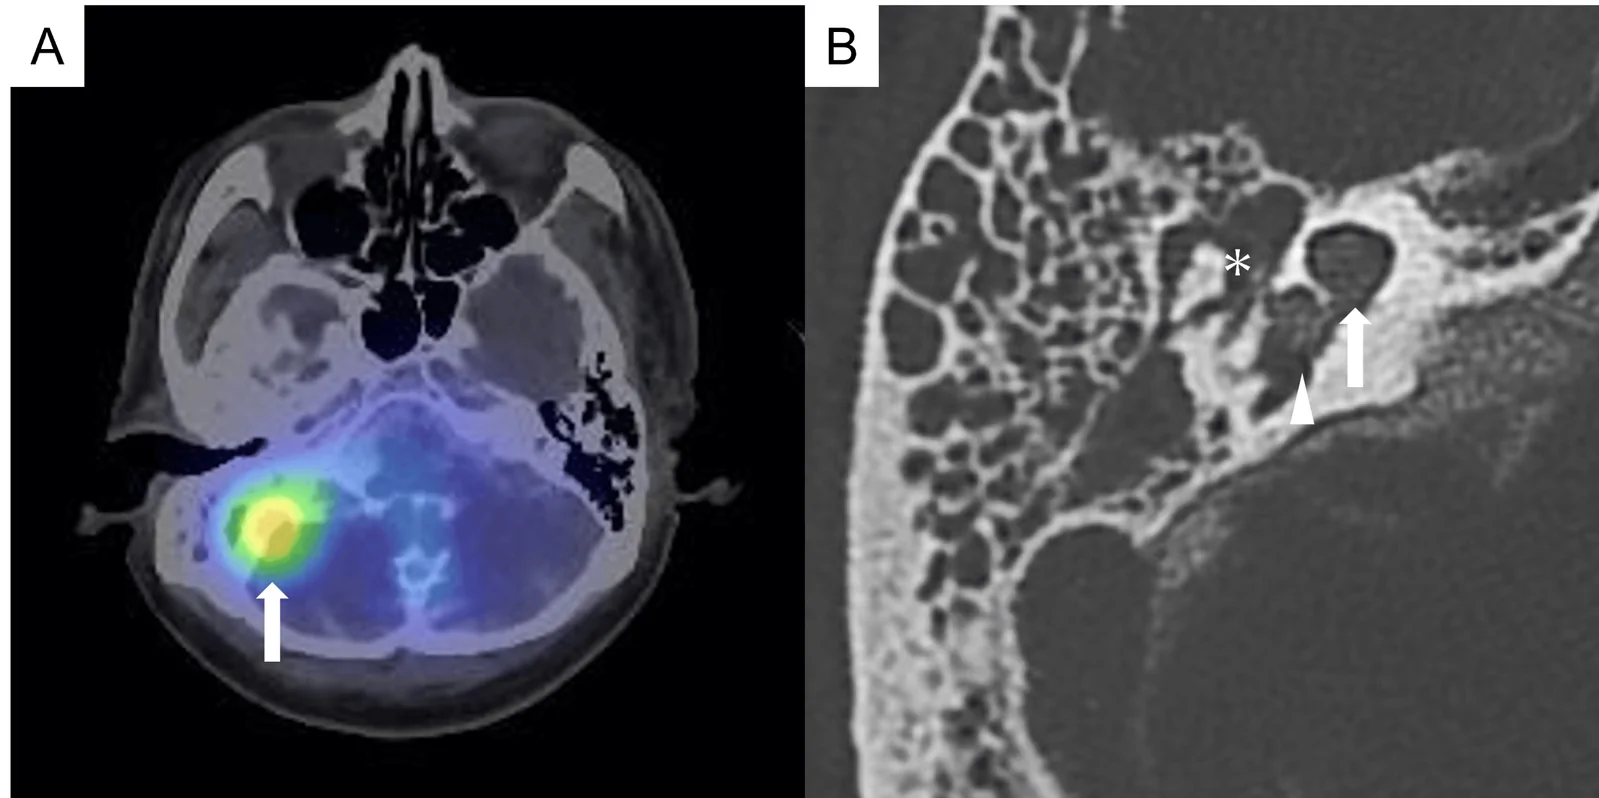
Cisternography. (A) Radionuclide cisternography: Images obtained six hours after intrathecal administration of ¹¹¹In-diethylenetriaminepentaacetic acid. Abnormal tracer accumulation was observed in the right mastoid cavity (arrow). (B) CT cisternography: Contrast leakage was observed extending from the internal auditory canal through the cochlea (arrow) and vestibule (arrowhead) into the middle ear cavity (asterisk).
